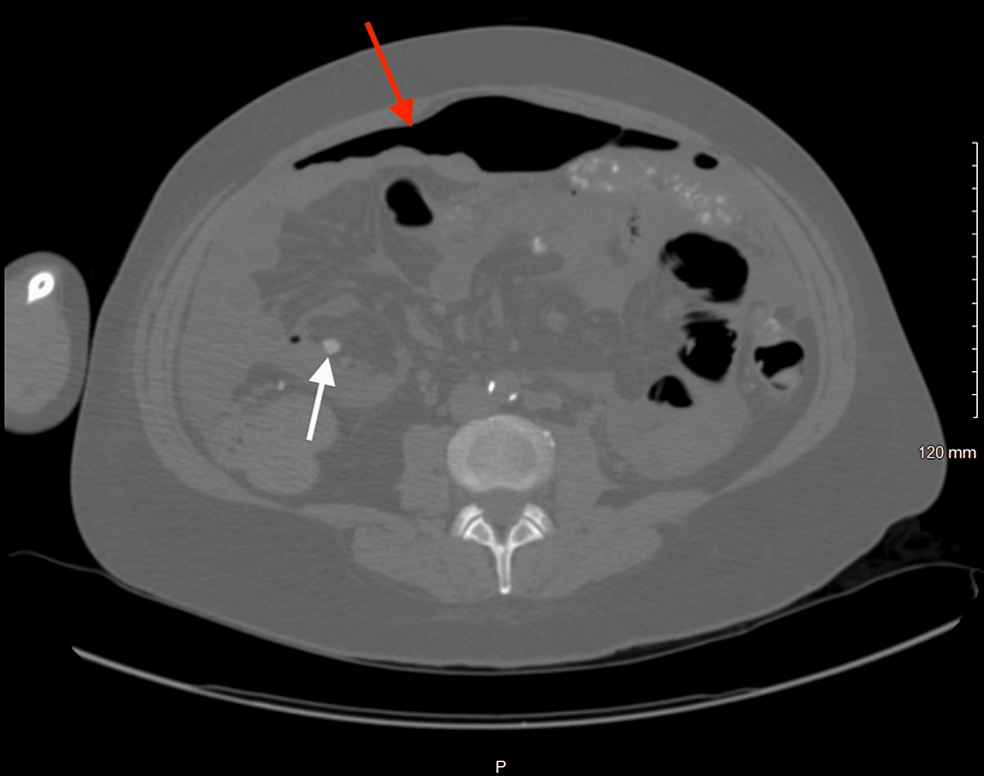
A CT of the abdomen and pelvis without IV contrast was performed on October 25, 2022 Impression noted bibasilar discoid atelectasis and/or atelectatic infiltrates. A moderate amount of pneumoperitoneum (red arrow) is worrisome for a ruptured viscus; it is of note that the patient’s last paracentesis was seven days ago. Moderate abdominal and pelvic ascites. Redemonstration of findings compatible with extensive peritoneal carcinomatosis (white arrow).
